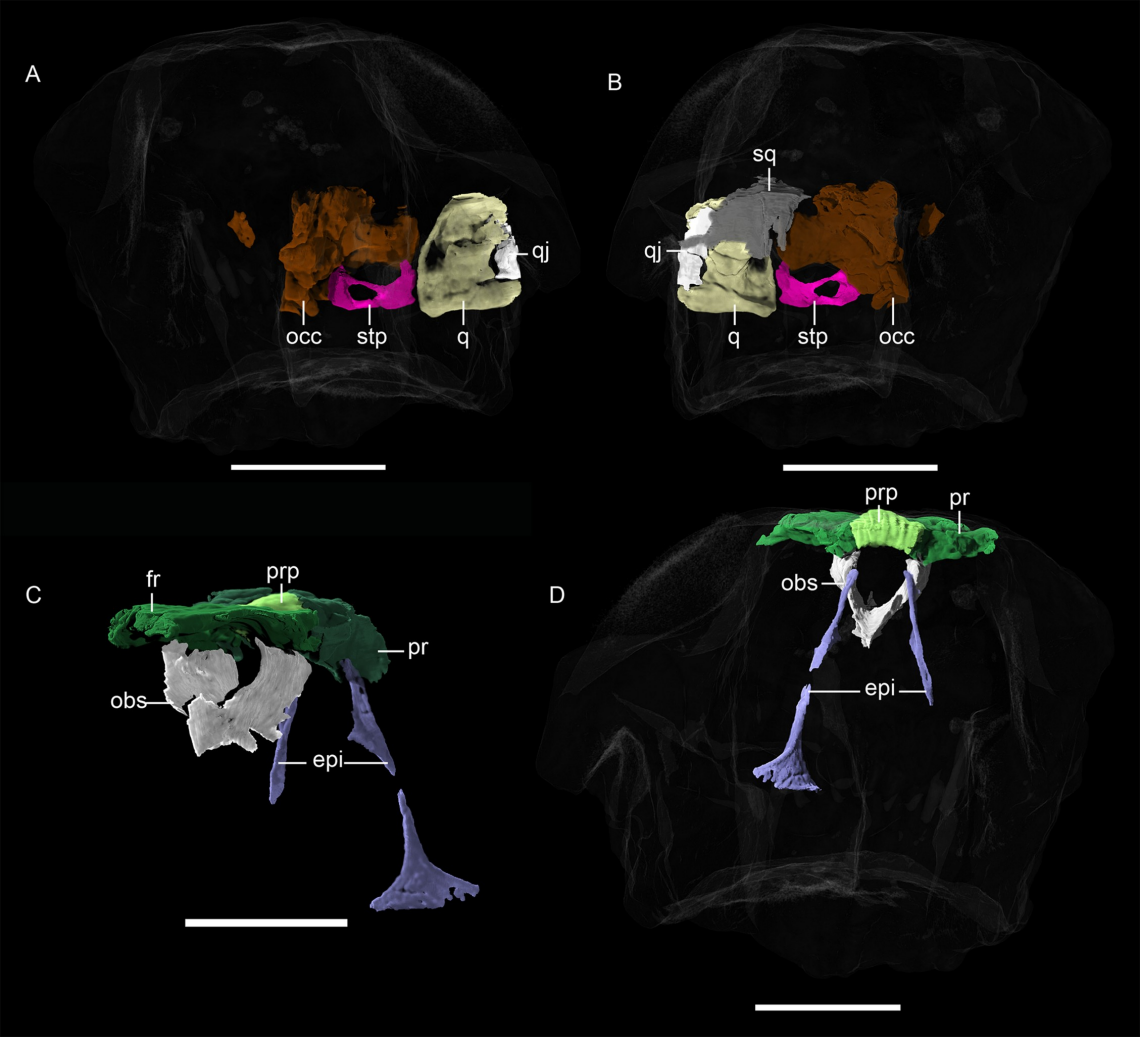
Neutron CT reconstruction of MB.R.999: occipital region from (A) anterior and (B) posterior; epipterygoid/orbitosphenoid from (C) anterolateral and (D) posterior. Abbreviations: epi, epipterygoid; fr, frontal; obs, orbitosphenoid; occ, fused occipital components; pr, parietal; prp, preparietal; q, quadrate; qj, quadratojugal; sq, squamosal; stp, stapes. Scale bars = 2 cm.
